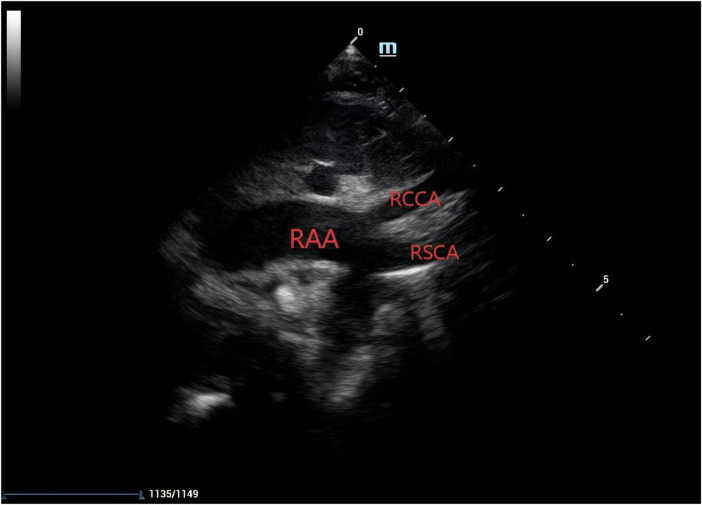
Postnatal echocardiographic view demonstrating two branches arising from the right aortic arch (RAA, right aortic arch; RCCA, right common carotid artery; RSCA, right subclavian artery).
